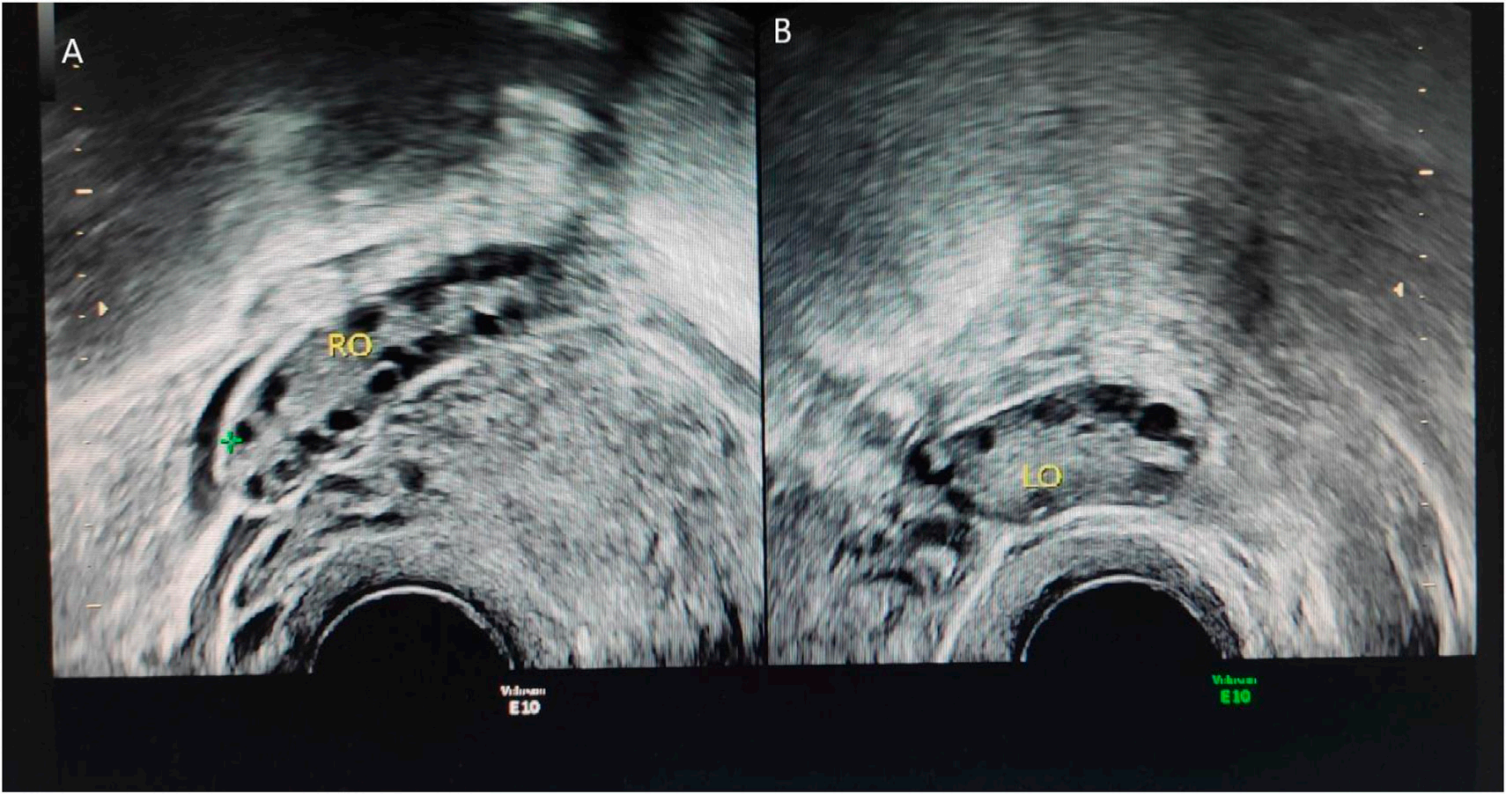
Ultrasound results of patient 4. Thin-slice CT of the adrenal glands demonstrates no abnormalities. (A) represents the right ovary of the patient. The right ovary is 22.4 × 9.9 mm, and approximately five follicles can be seen in the right ovary. The larger diameter is approximately 8.6 mm, and the smaller diameter is approximately 4.0 mm. (B) represents the left ovary of the patient, the size of the left ovary is 24.0 × 9.7 mm, and approximately four follicles can be seen in the left ovary, the larger diameter is approximately 7 mm, and the smaller diameter is approximately 4.6 mm indicates large volume of both ovaries accompanied by more follicles.
